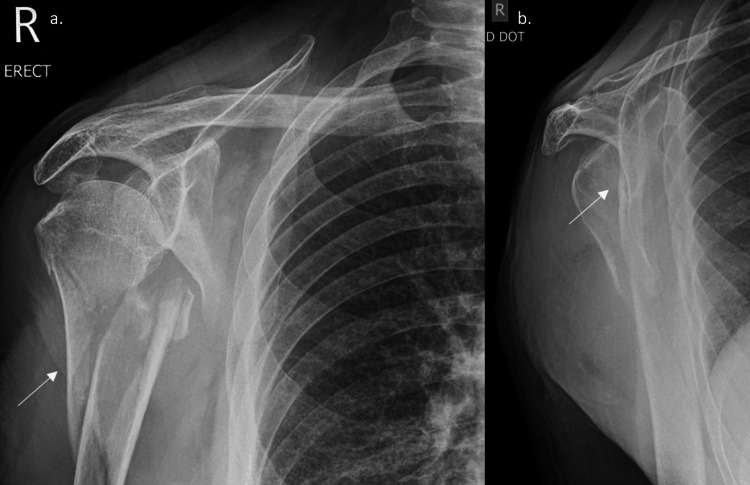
Initial radiographs showing a comminuted spiral fracture of the proximal humerus: (a) anteroposterior chest and (b) Y-view of the shoulder The white arrows indicate the fracture.
